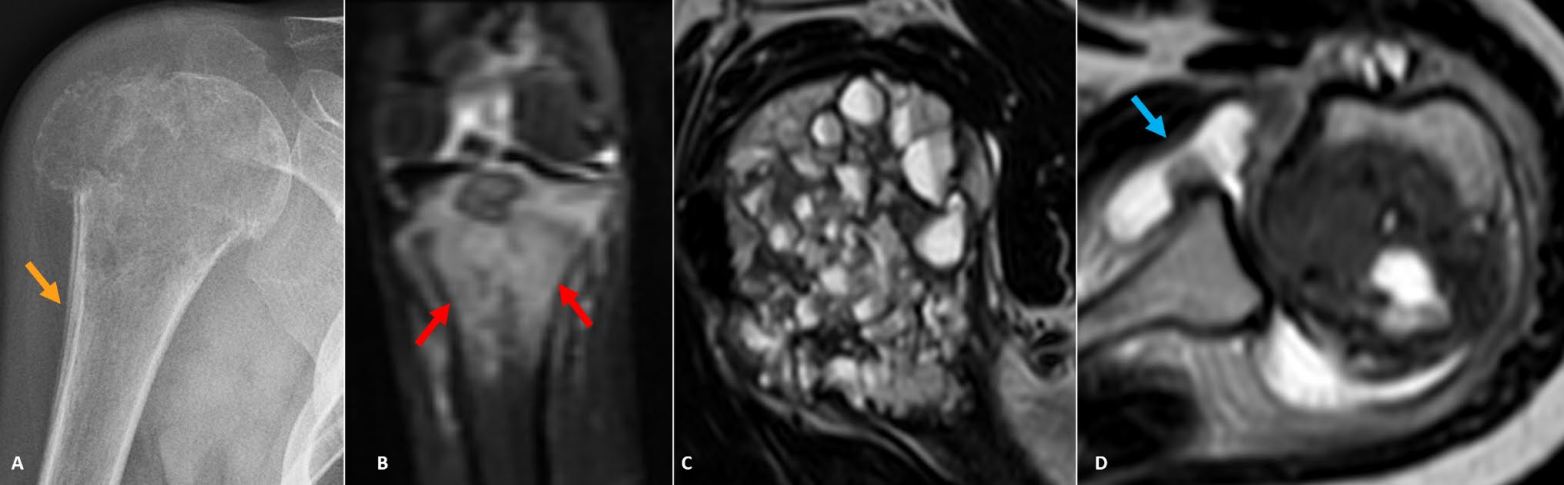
Aggressive imaging features in chondroblastoma. A Cortical breach, periostitis, pathologic fracture—radiograph of the right humerus with greater tuberosity chondroblastoma demonstrating cortical breech and linear periosteal reaction (arrow) along humeral meta-diaphysis. Also note minimally displaced pathological fracture. B Exuberant edema—coronal STIR MRI image in a proximal tibial chondroblastoma demonstrating exuberant marrow edema (red arrows) disproportionate to lesion size. C Fluid–fluid levels—sagittal T2W MRI image showing innumerable fluid–fluid levels in a humeral chondroblastoma. D Joint effusion—T2 intermediate to hypointense humeral lesion with articular extension and moderate joint effusion (arrow)
